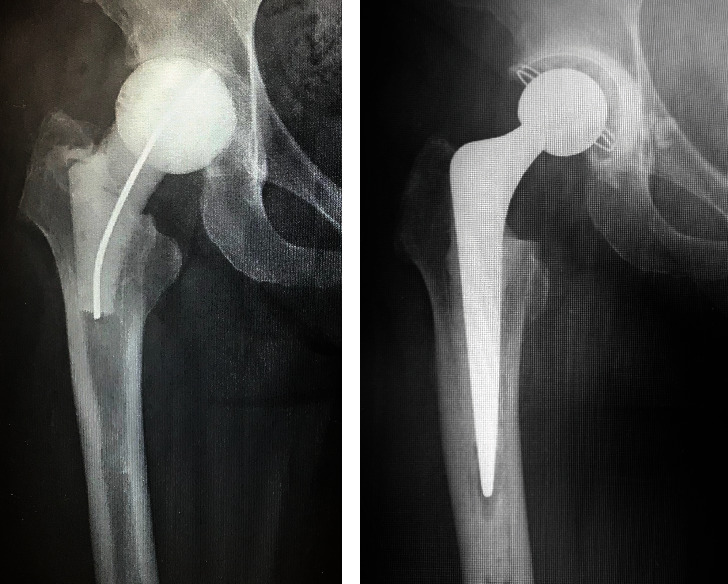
Postoperative plain radiograph. (a) Antibiotic cement mold was inserted in first surgery. (b) Cemented THA was performed in second surgery.
